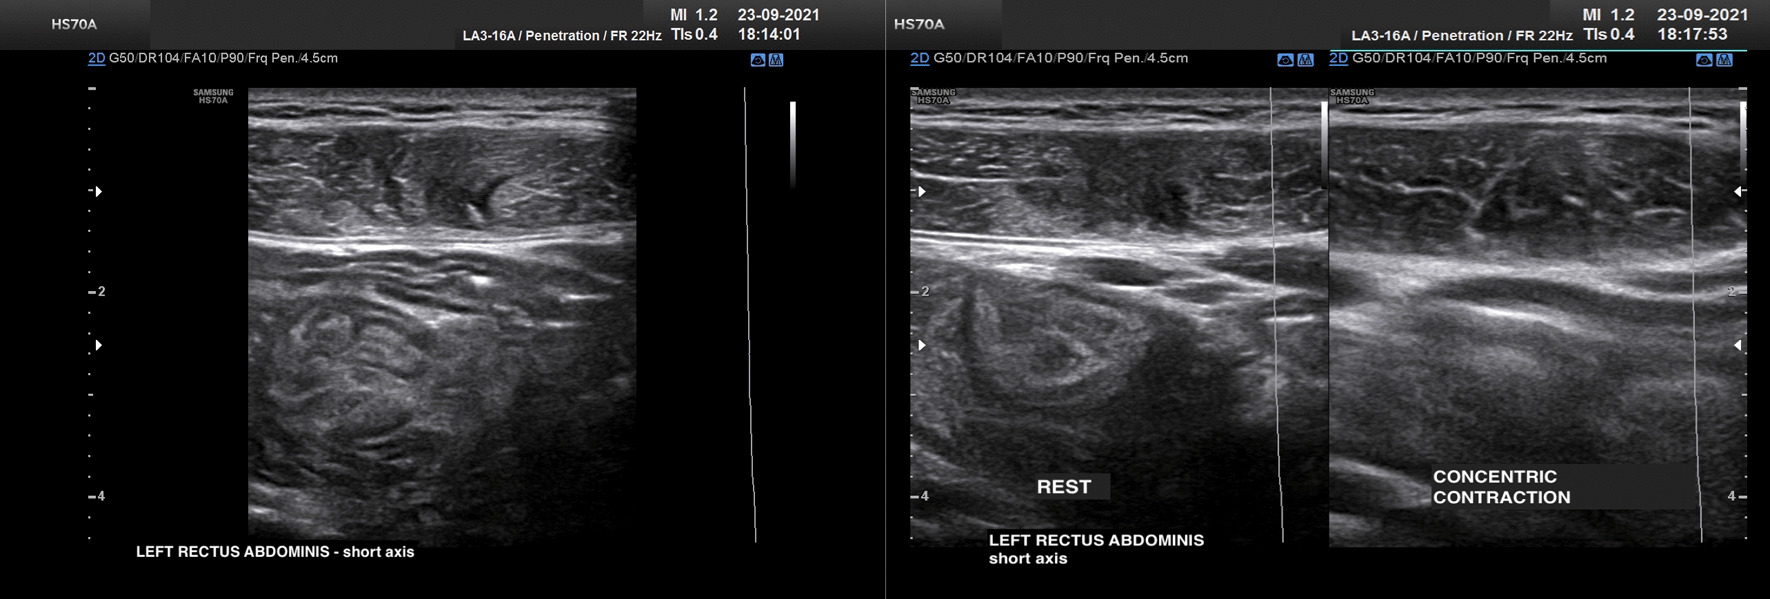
The ultrasound shows the apposition of tissue with varying degrees of echogenicity (from hypoechoic to iso-hyperechoic), which completely rehabilitates the area of discontinuity. Power Doppler hypervascularization patterns are not observed. The execution of the functional tests of concentric activation of the rectus abdominis (crunch and leg raise) highlights regular tensioning of the repair tissue with residual, minimal response to reduced resistance in the context of the hypoechoic tissue alone. The finding depicts the results of II-degree stabilized lesion (3B according to the Munich consensus 2012) of the left rectus abdominis in the stabilization phase
